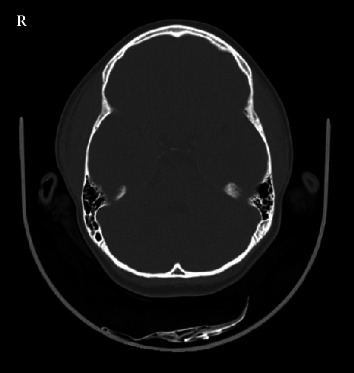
Computed tomography of the head. Axial cut showing thickening of the right parietotemporal epicranial soft tissues.
